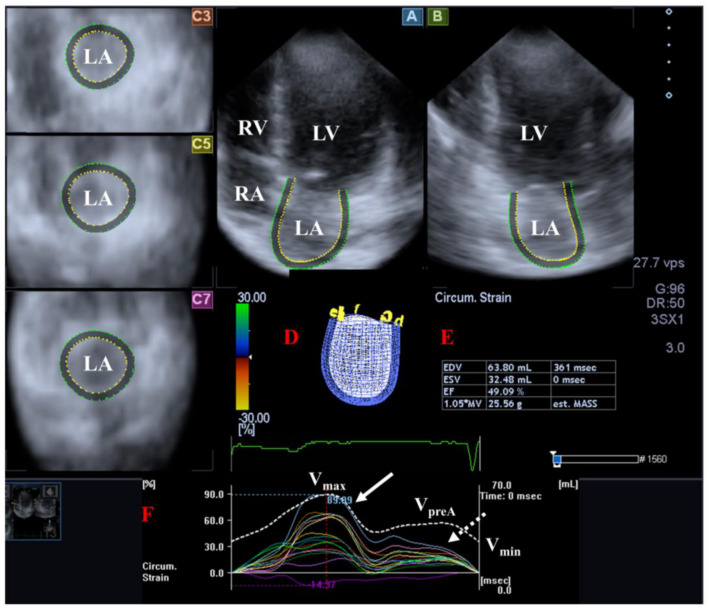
Examination of the left atrium [LA] by three-dimensional [3D] speckle-tracking echocardiography. Following echocardiographic data acquisitions, the following typical views are created: (A) Apical 4-chamber and (B) two-chamber longitudinal views and (C3,C5,C7) cross-sectional views at basal, midatrial and superior levels, respectively. LA can be easily detected alongside other heart cavities including the left ventricle [LV] and the right atrium [RA] and ventricle [RV]. A number of other details were also presented including (D) 3D cast, (E,F) maximum [V_max_], preatrial contraction [V_preA_] and minimum [V_min_] volumes of the LA and curves representing changes in volumes and strains of LA over time. Reservoir [peak] and active contraction LA strains are represented by white and dashed white arrows, respectively.
